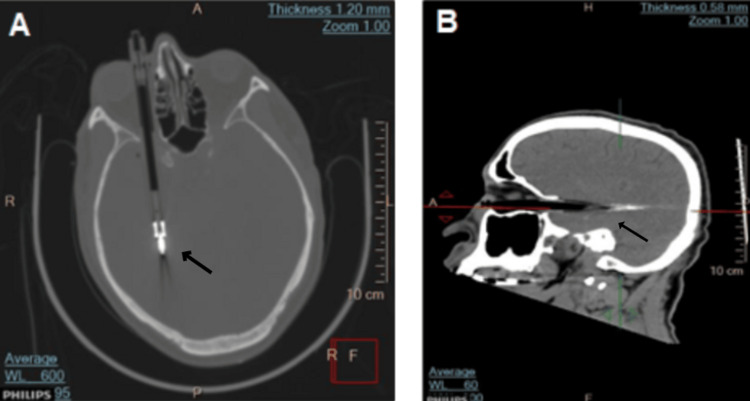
CT of the head outlining the extent of pen insertion. A. Axial CT scan of the head providing a more detailed view of the placement of the ballpoint pen through the right orbit and brain. B. Sagittal CT view of the head highlighting the level of penetration of the ballpoint pen through the right orbit and traversing into the right temporal lobe.
